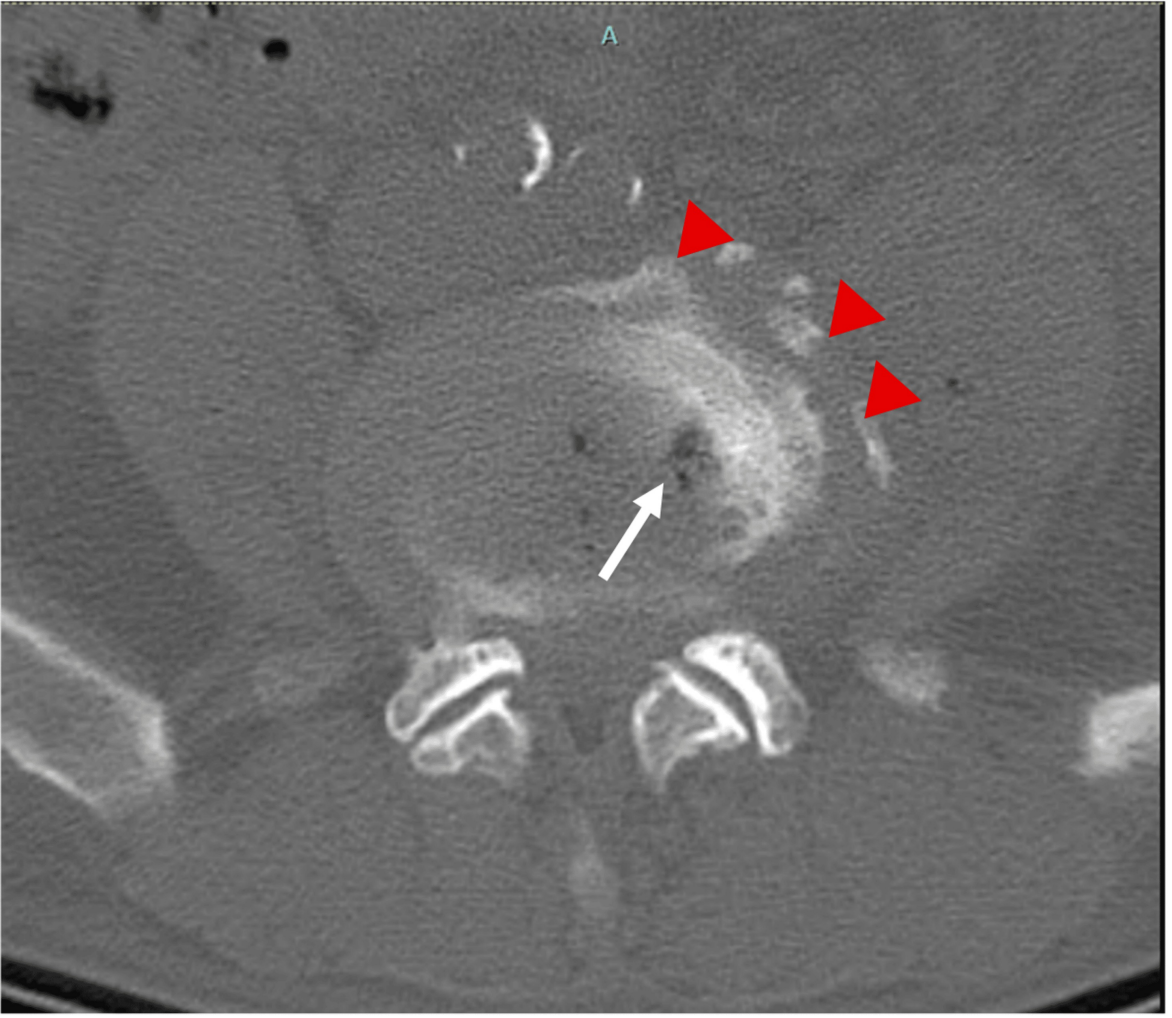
Non-contrast lumbar CT Axial view at the level of L4-L5 showing destruction and fragmentation of the vertebral body (red arrowheads), findings suggestive of an infectious process. Gas within the disk space may occasionally occur with infection (white arrow), although it is less common. CT - Computed tomography
